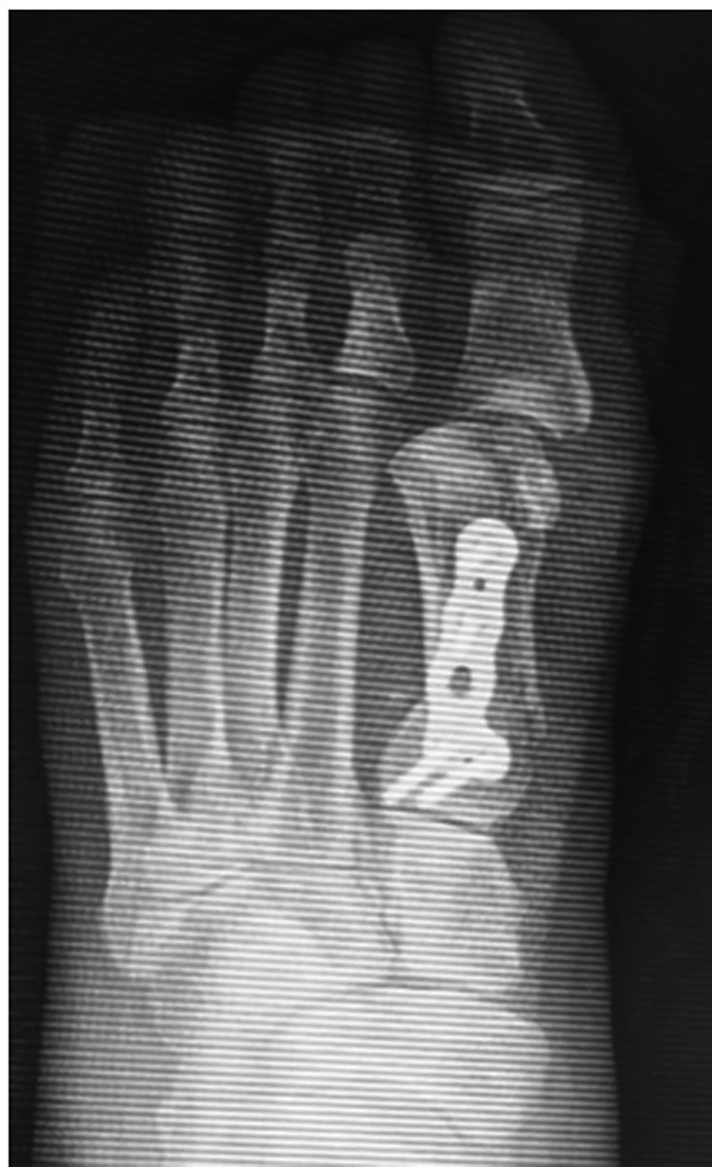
Radiograph showing incongruency of the first metatarsophalangeal joint due to overcorrection during surgery for hallux valgus, leading to hallux varus.
